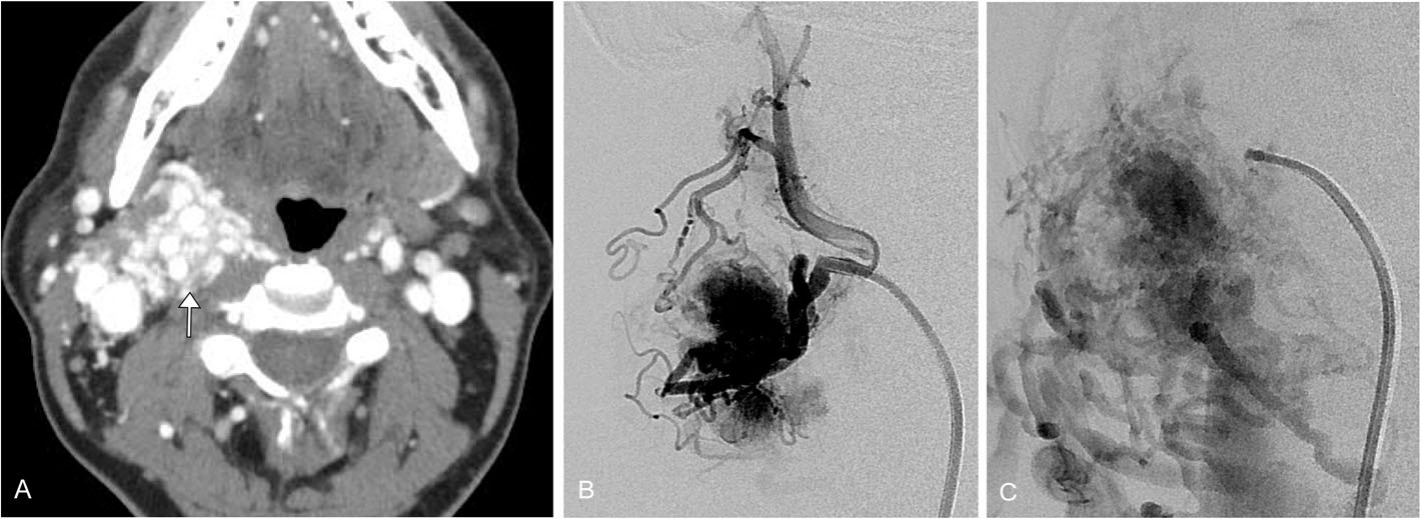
Arteriovenous vascular malformation. A 50-year-old male with right neck fullness. a Contrast-enhanced axial CT shows a tangle of serpiginous vessels in the right deep neck (arrow). b Conventional angiogram with injection of an external carotid artery dominant feeder shows brisk enhancement of the nidus. c Delayed angiographic image shows multiple draining venous vessels
